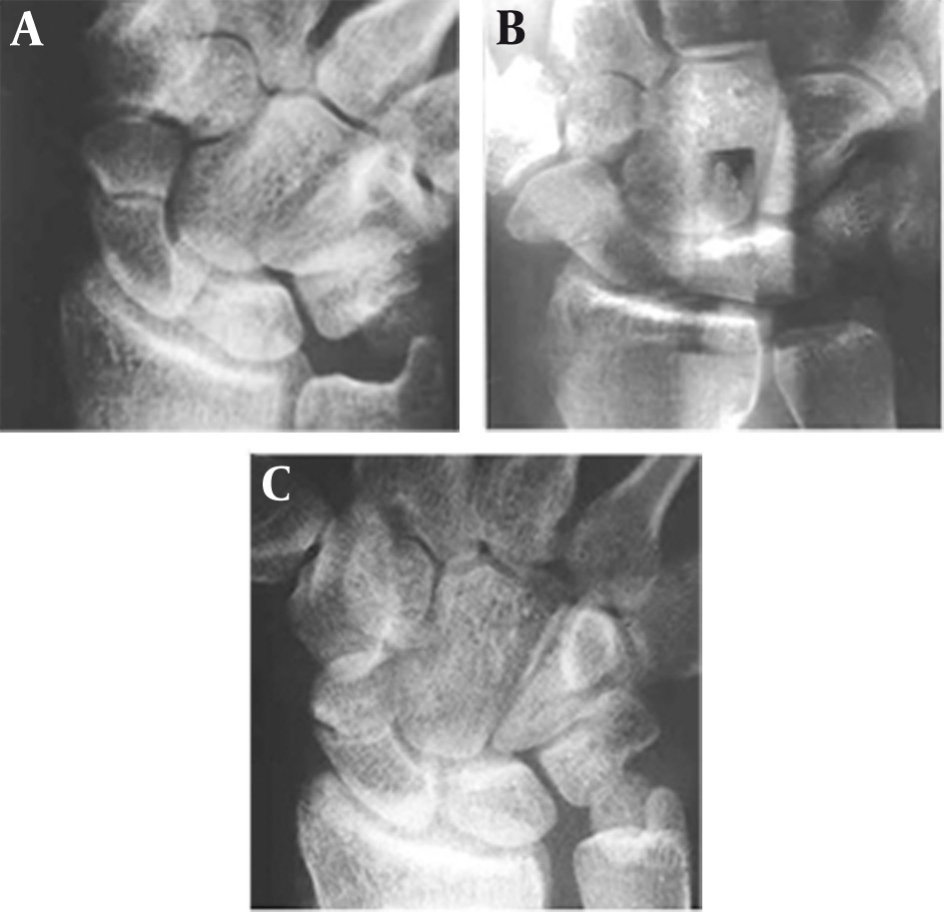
A 21-year-old man with wrist trauma. Conventional and panoramic radiographs were performed 10 weeks after injury because of the doubtful union of scaphoid fracture. A, Initial conventional radiograph shows doubtful scaphoid union. B, Panoramic radiograph shows the fracture partially united. C, Conventional radiograph obtained four months after trauma shows the fracture partially united, as seen in B.
